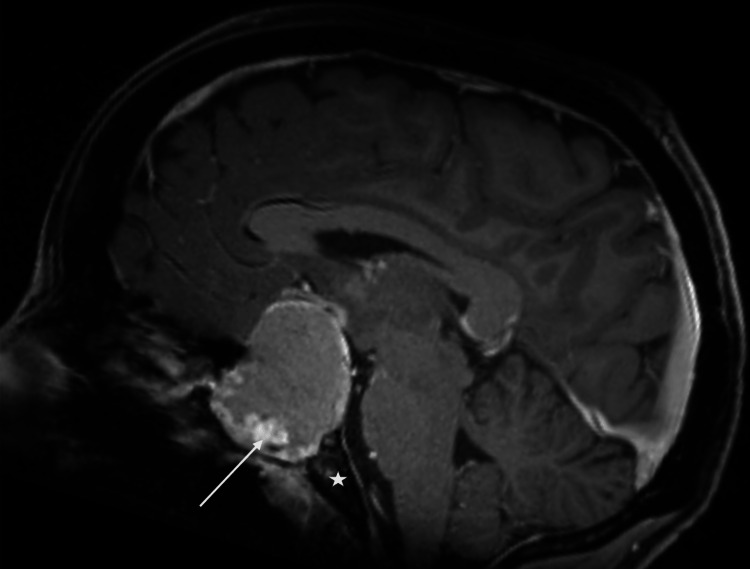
Sagittal T1-weighted post-contrast magnetic resonance imaging. Sagittal T1 post-contrast magnetic resonance image demonstrating a suprasellar lesion with enhancing nodular components (arrow). Clivus is represented by the star.
